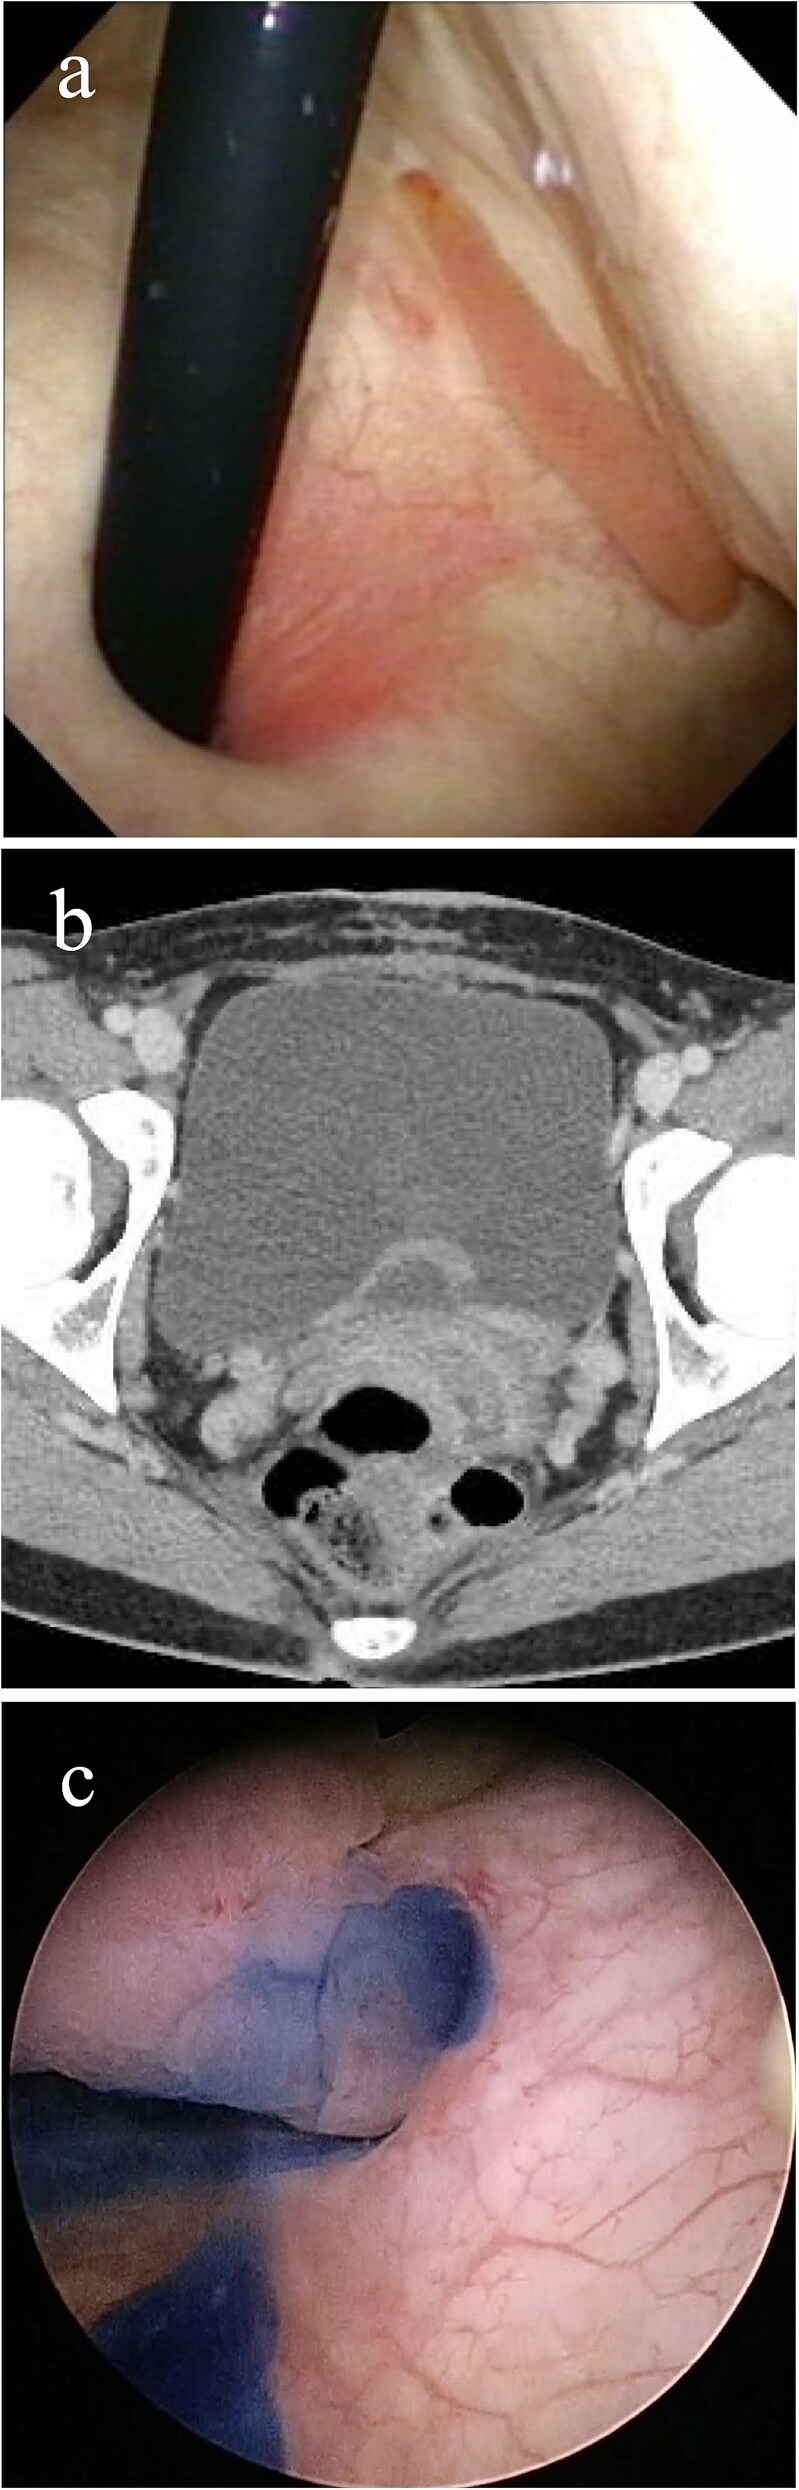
Imaging study findings. (a) Cystoscopy finding. Cystoscopy revealed that a tumor protruded from the left ureteral orifice into the bladder lumen, showing serpentine locomotion with respiratory movements. (b) Contrast enhanced computed tomography finding. Soft tissue shadow was detected around the left ureteral orifice, revealing a long thin tumor. (c) Indigo carmine was excreted through the left orifice that is occupied by the tumor.
